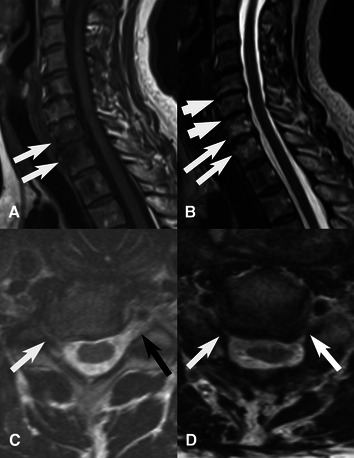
MRI of three different patients. (A,B) T1W-, and T2W sagittal images show Modic type 1 changes with bone marrow oedema around C7-Th1-disc space (thin long arrows) and moderate disk degeneration C5–C6 and C6–C7 (thick short arrows). (C) T2W axial image shows right sided foraminal stenosis C6–C7 with severe narrowing of neural foramen compression (white arrow) and C7-nerve root. Black arrow shows normal left neural foramen. (D) T2W axial image shows bilateral foraminal stenosis at C5–6 level with C6-nerve root compression.
